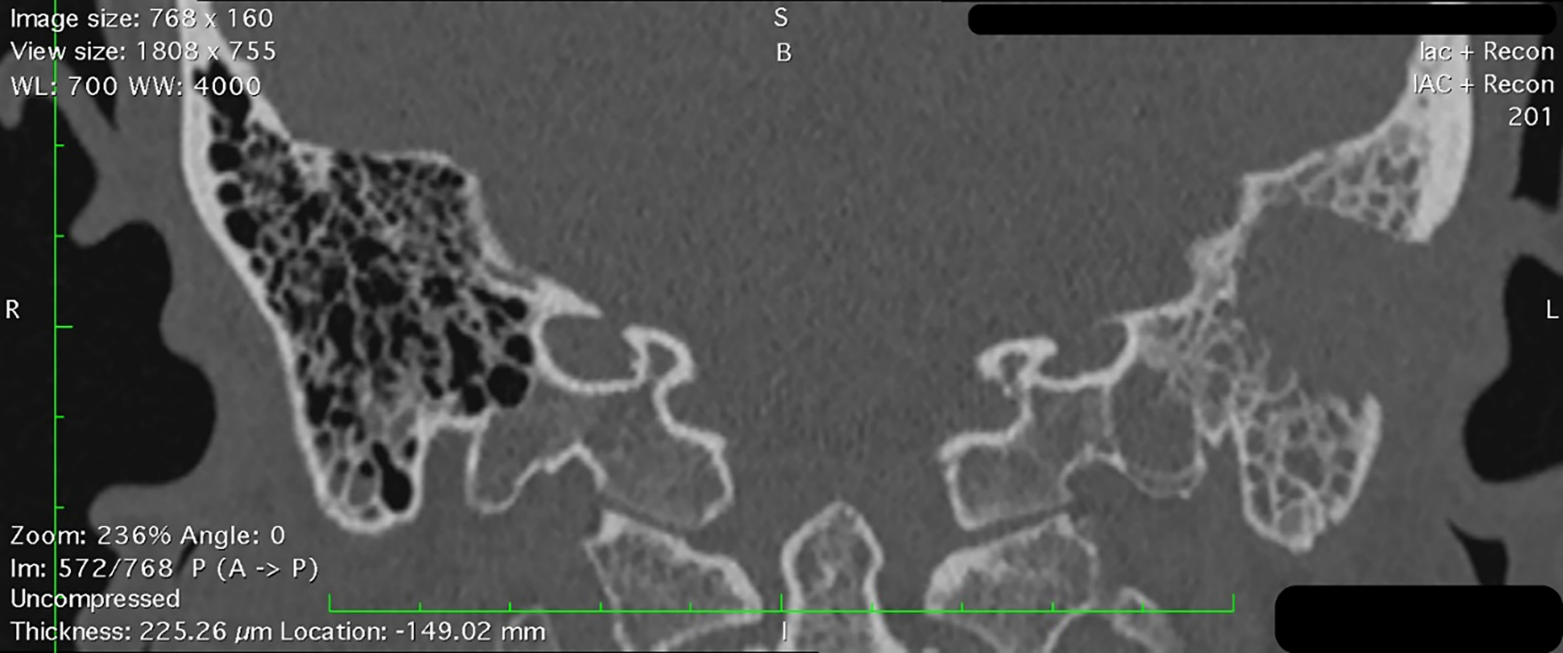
Head computed tomography, post‐mastoidectomy. Computed tomography reveals persistent opacification in the remaining mastoid cells on the left and partial opacification of the right mastoid cells.
